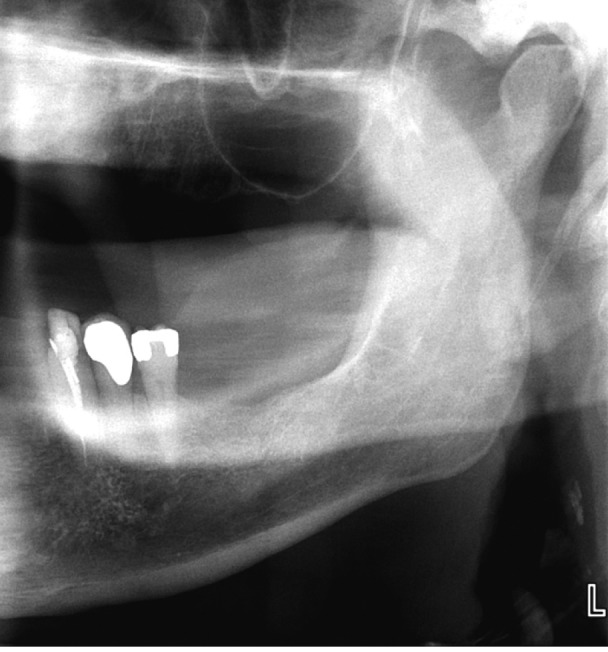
A cropped panoramic radiograph of the same individual in Figures 1 and 2. Note the two calcified carotid artery atheroma superior to the prominent semi lunar shaped epiglottis and the letter “L” marker.
